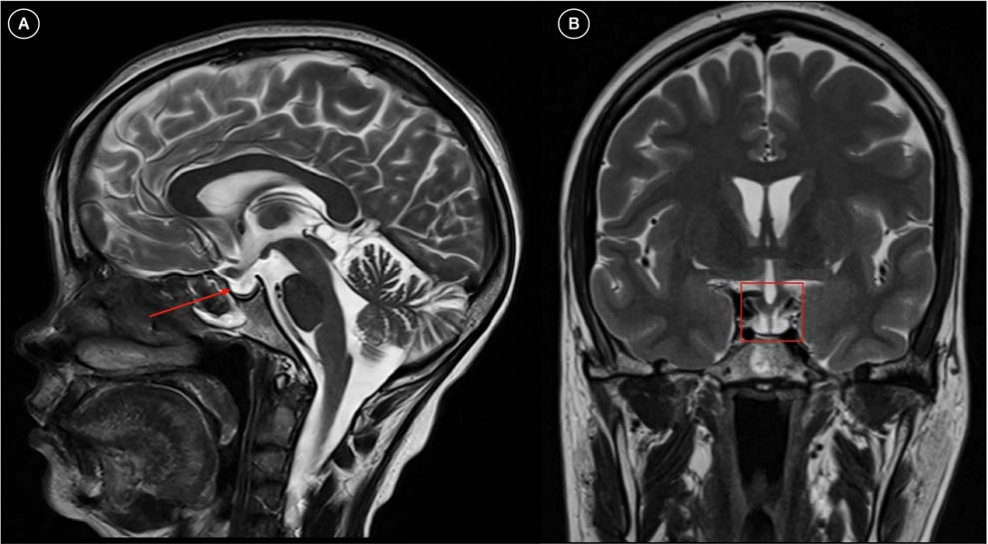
T2-weighted magnetic resonance imaging shows (A) a normal-appearing sella filled with cerebrospinal fluid (sagittal view; red arrow) and (B) the infundibular stalk traversing the empty cerebrospinal fluid–filled sella without displacement (coronal view; red box).
